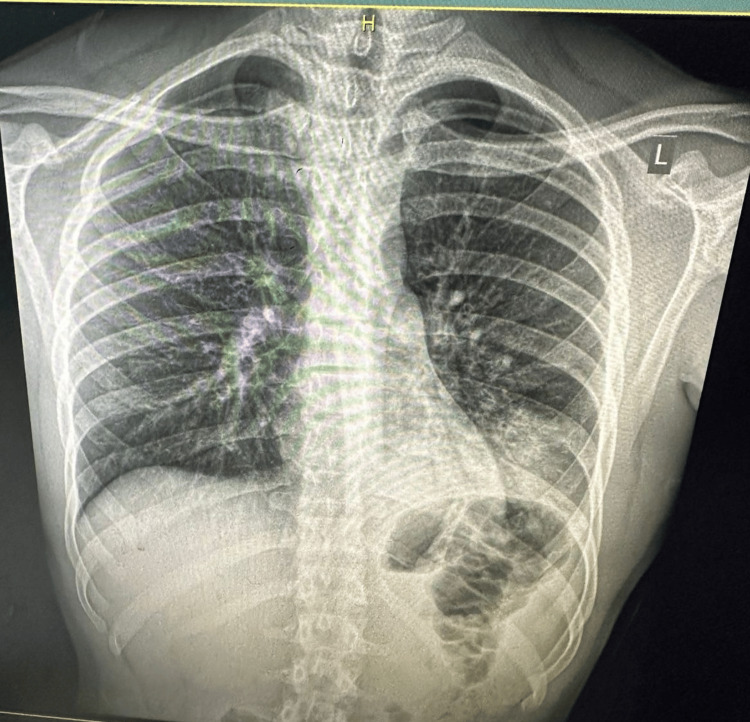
Anteroposterior view of chest X-ray on admission: bilateral, predominantly lower zone patchy airspace/interstitial infiltrates, left > right, with a patchy consolidation in the left lung base.
